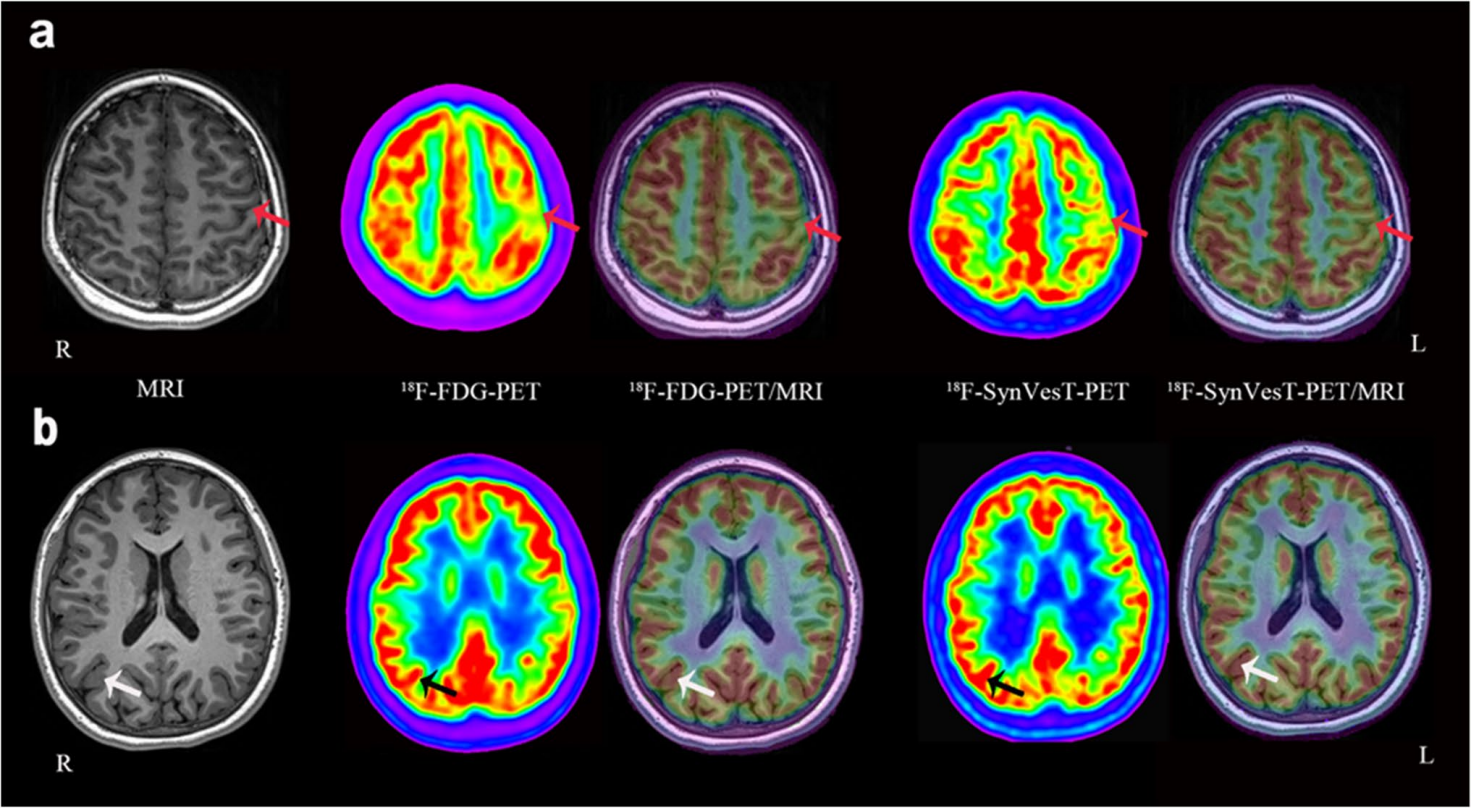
MRI, ^18^F-FDG, and ^18^F-SynVesT-1 PET/MRI images of the 2 FCD IIa patients. FCD IIa in two patients could not be detected by the initial visual assessment on ^18^F-SynVesT-1 PET. a The lesion in patient 4 was detected after superimposition of ^18^F-SynVesT-1 and ^18^F-FDG PET to MRI images, with the lesion located in the left frontal lobe (which displayed a small AI, 11.00%) (red arrows). b The lesion in patient 9 was not localized by the three neuroimaging methods. It was finally confirmed that the lesion was located in the right occipital lobe (white and black arrows). We assumed that FCD IIa might have a nonsignificant reduction in SV2A with ^18^F-SynVesT-1 PET
